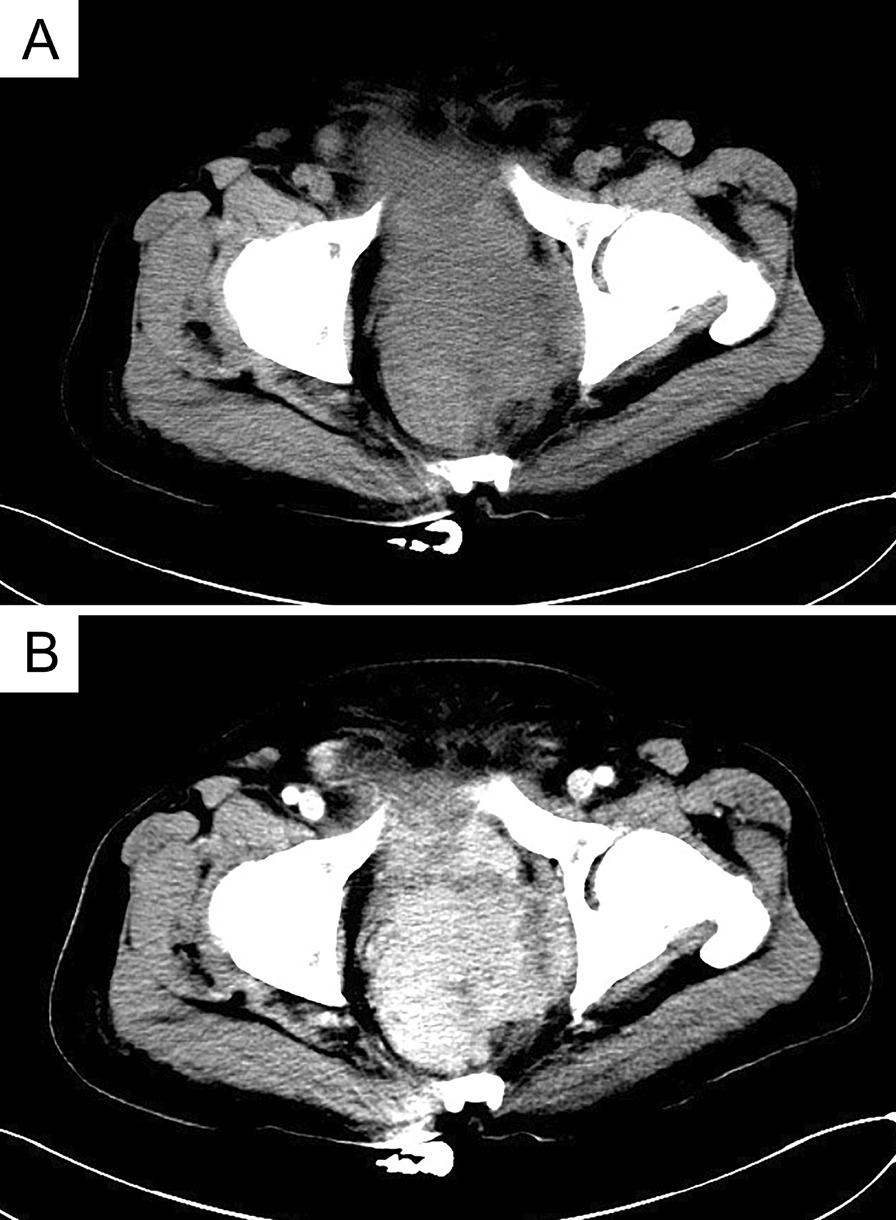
A CT scan showing an abnormal morphology of the prostate and bilateral seminal vesicles, with a large mass in the prostate area with unclear boundaries with the posterior wall of the bladder and rectum. B Enhancement scan showing significant inhomogeneous enhancement of the mass in the arterial phase
